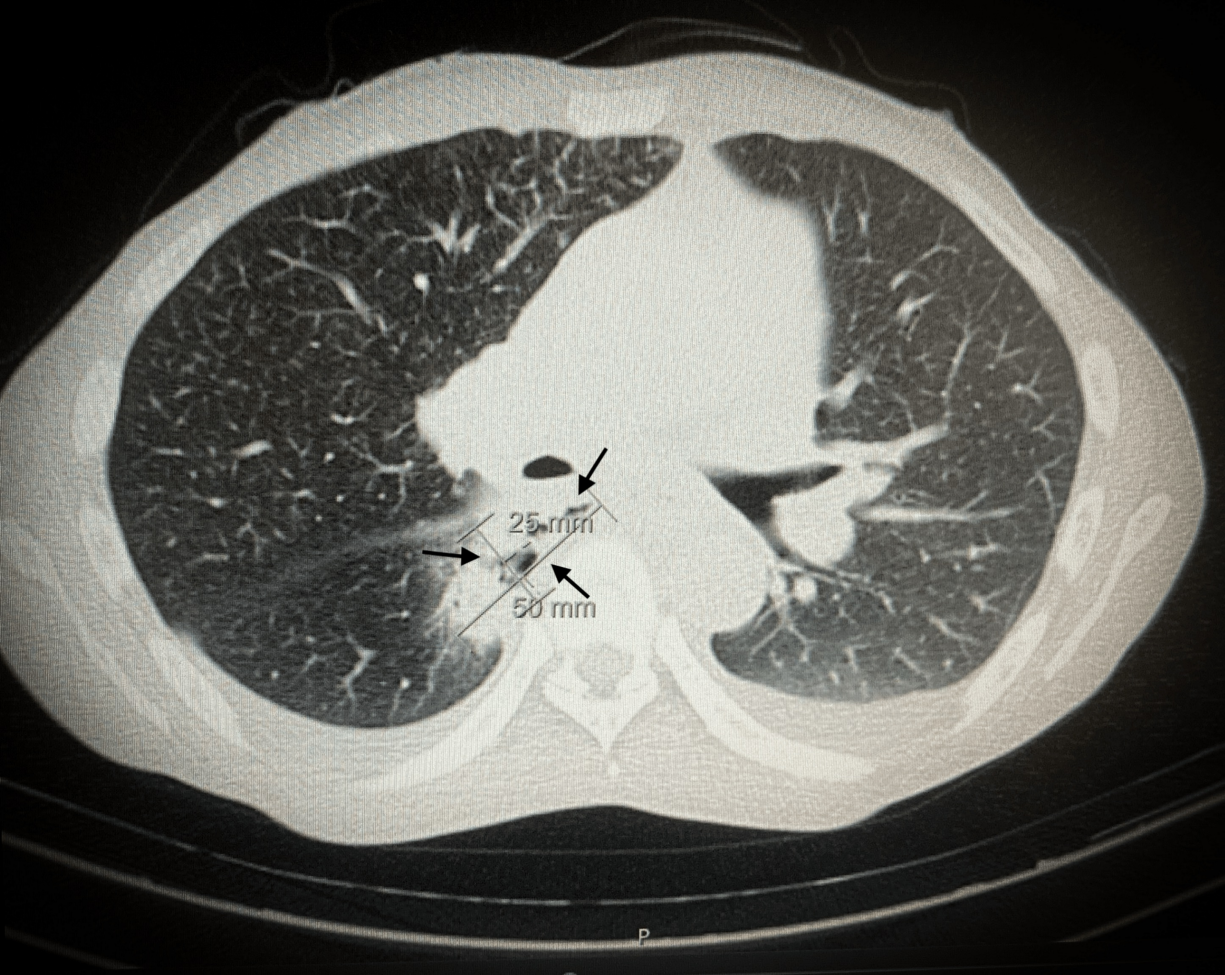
CT scan showing a fistulous tract extending towards the right-sided paravertebral collection containing both fluid and air (arrows) CT: computed tomography
